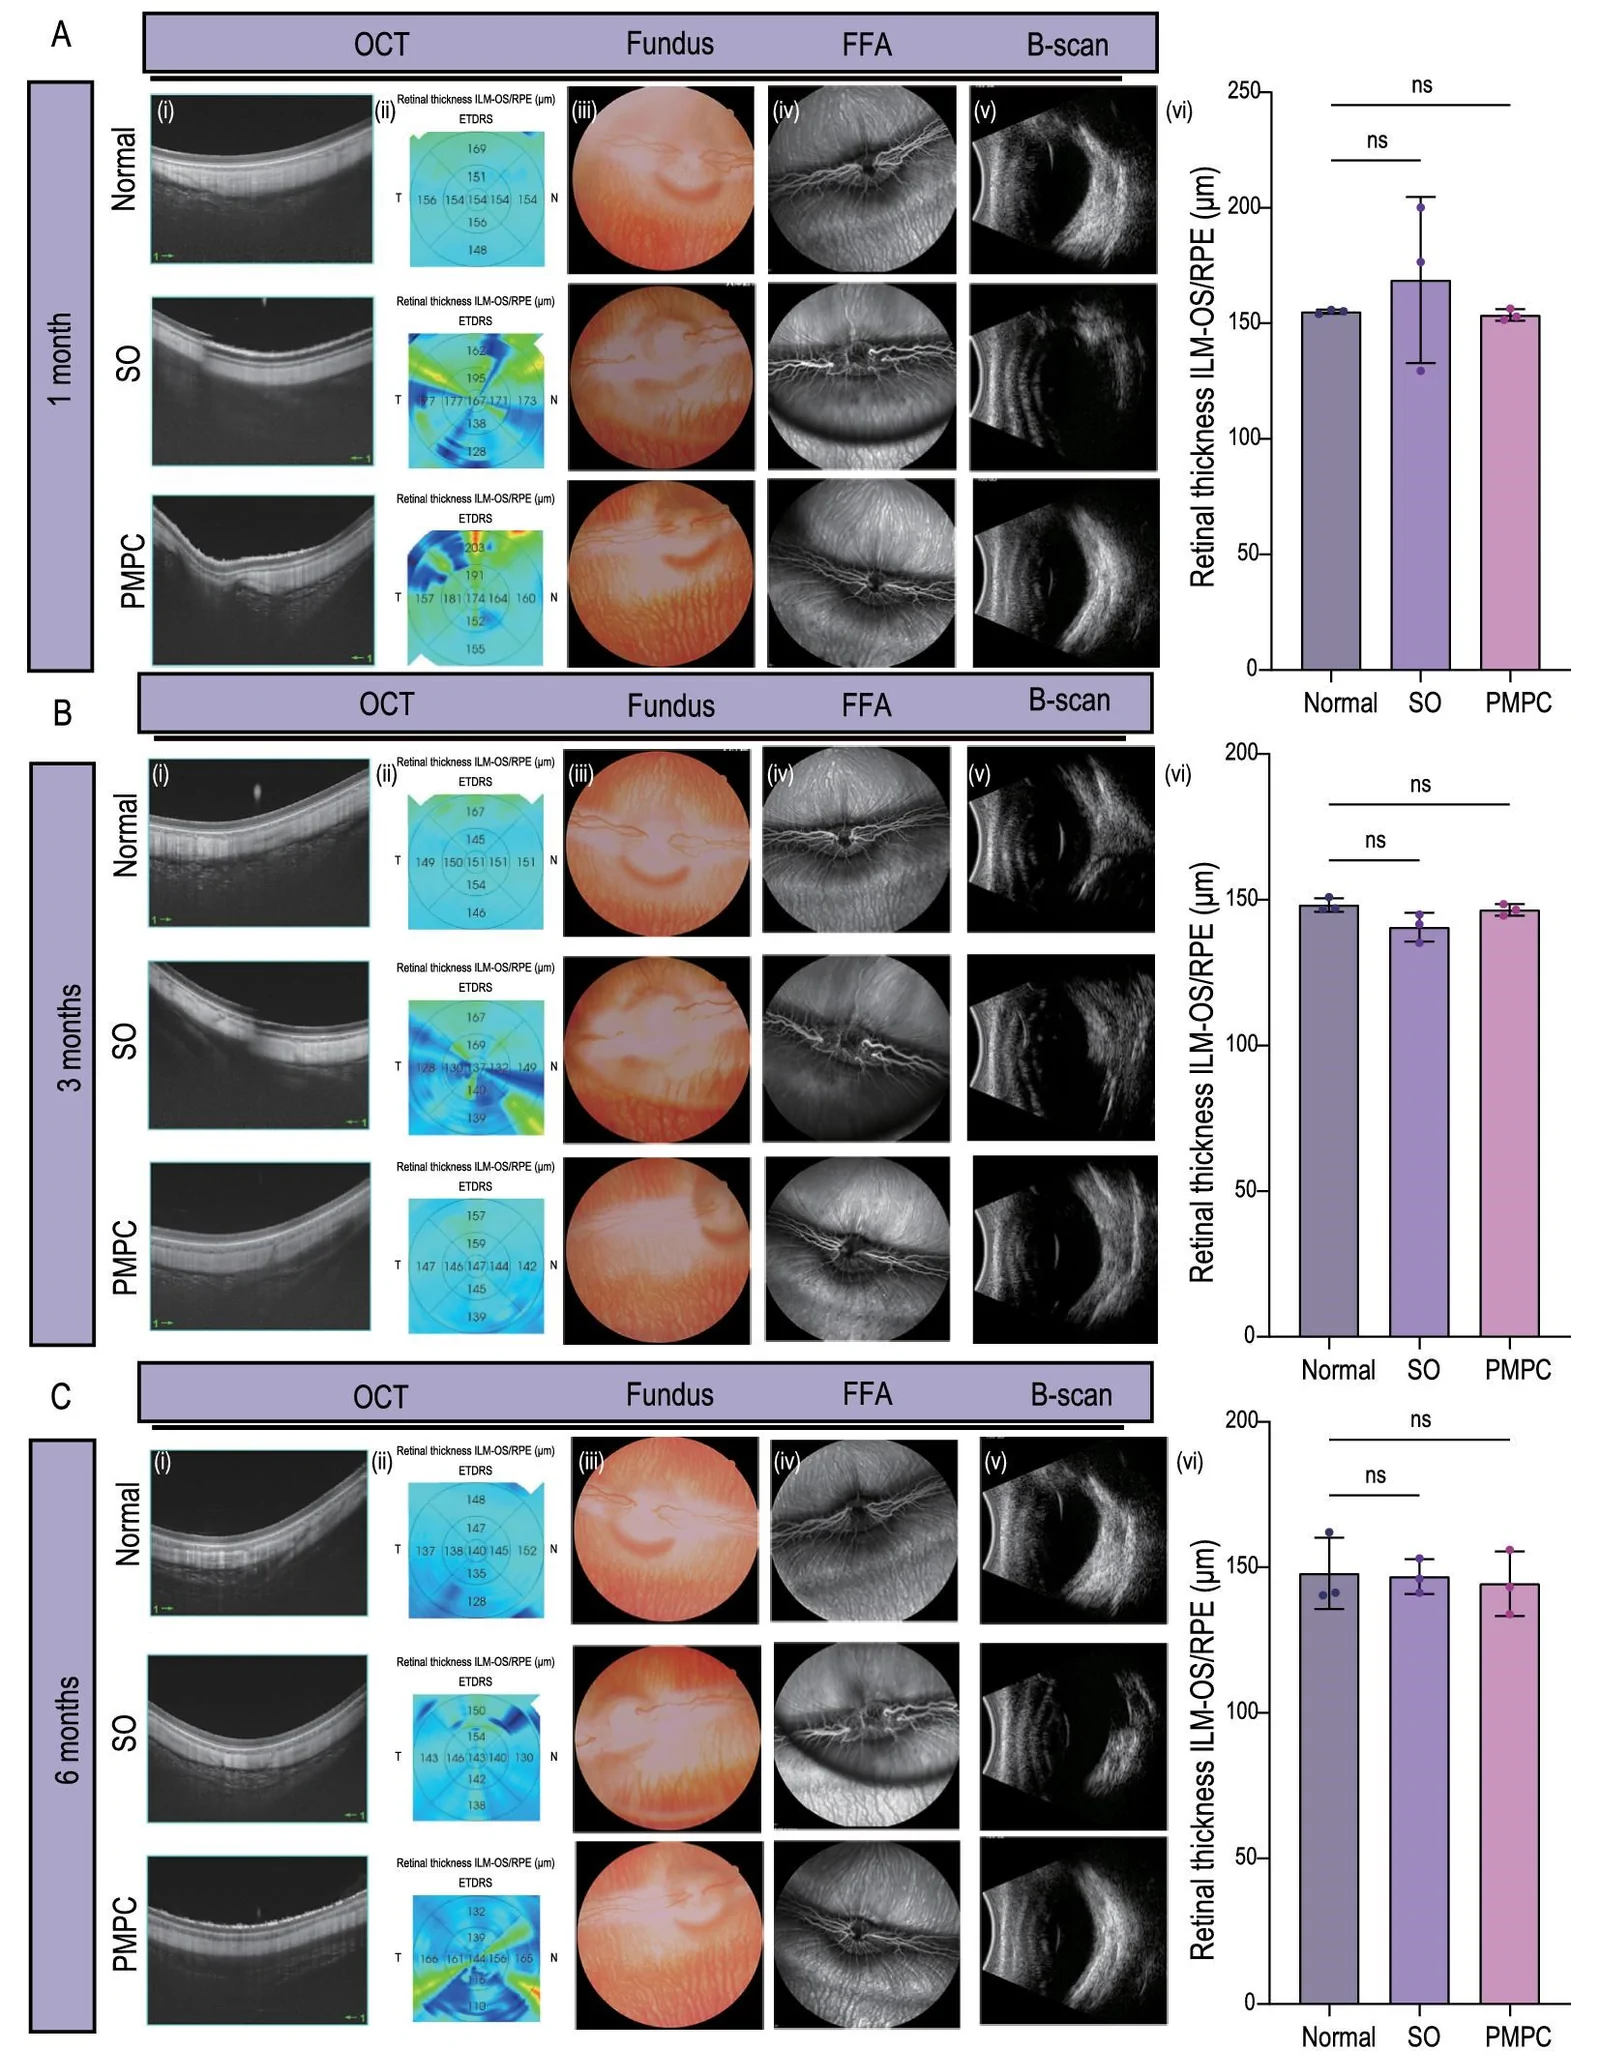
Representative images of OCT, fundus, FFA and B-scan. (A–C) Representative images of OCT, fundus, FFA and B-scan at 1 month (A), 3 months (B) and 6 months (C). (i–v) Representative retinal OCT images (i), retinal thickness map (ii), fundus images (iii), FFA images (iv) and B-scan ultrasonography images (v). (vi) Statistical analysis of retinal thickness. Data are presented as mean ± SD (*n* = 3). *P* values were calculated using one-way ANOVA followed by Tukey’s post hoc test. ns, not significant.
